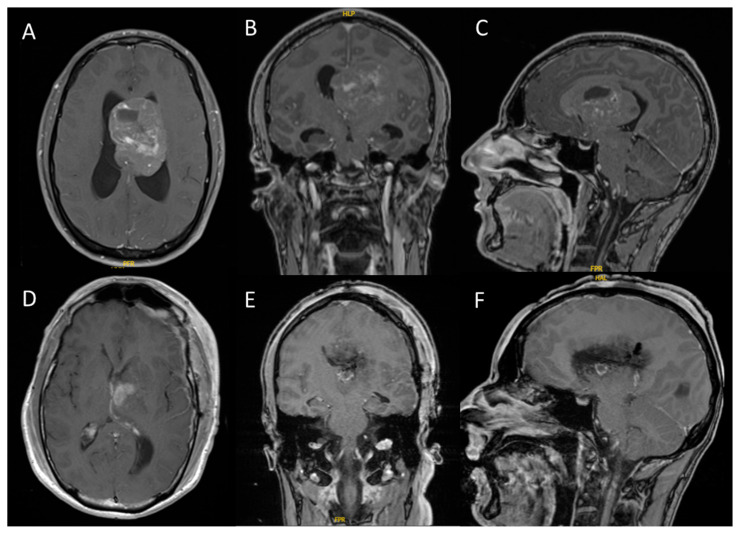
Intraventricular CN with hydrocephalus ((A–C): preoperative T1-weighted MRI with gadolinium contrast; (D–F): postoperative T1-weighted MRI with gadolinium contrast). These representative images are from case 1, a 31-year-old male who presented with headaches and cognitive deficits over the previous eight weeks. All tumors demonstrated contrast enhancement on MRI, with some cases exhibiting diffuse enhancement.
